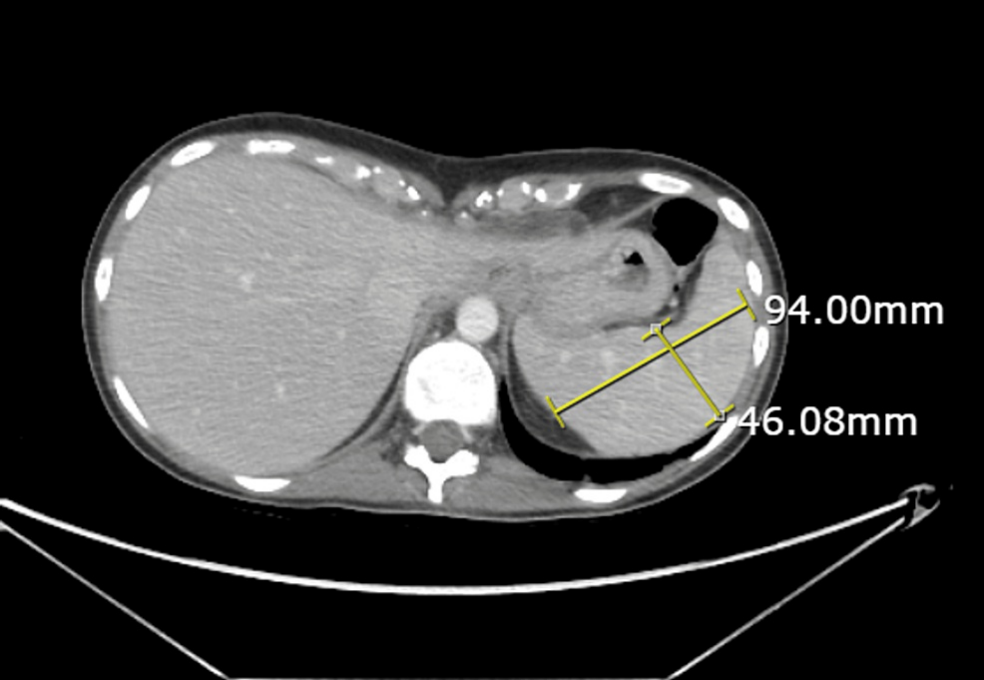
CT abdomen showing enlarged spleen
